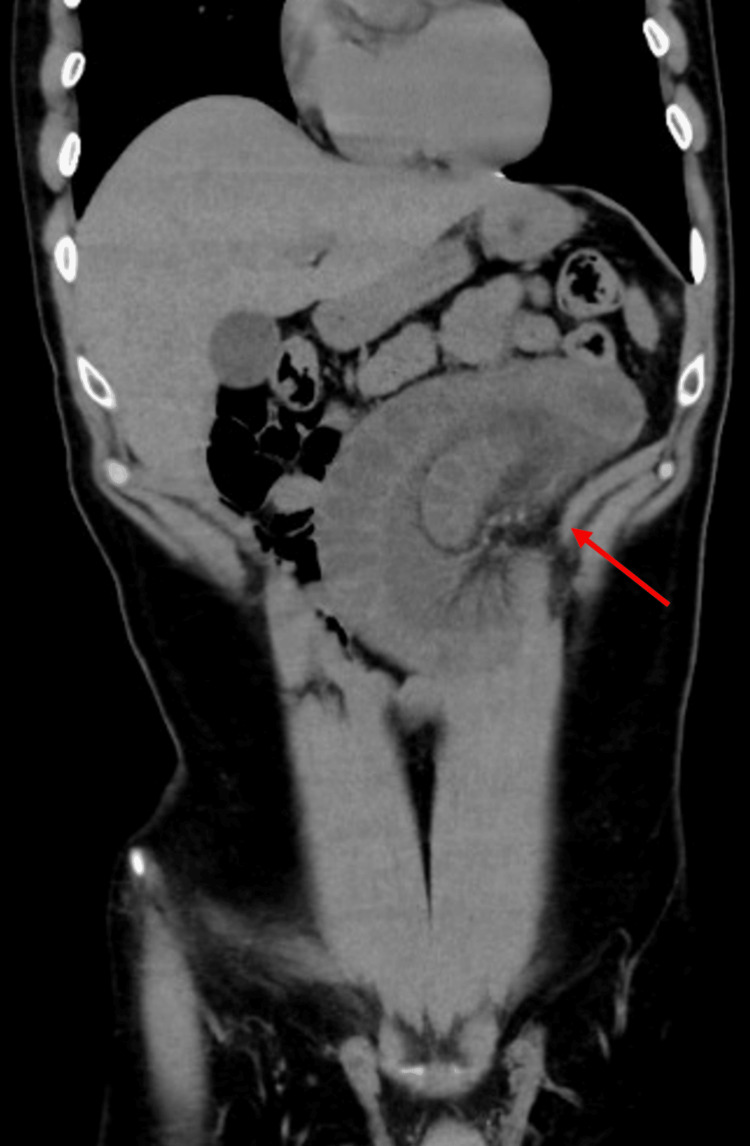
A contrast-enhanced CT scan of the abdomen and pelvis reveals a cluster of hypo-enhancing jejunal loops (indicated by arrow) measuring about 3 cm, with mild diffuse mural thickness.
